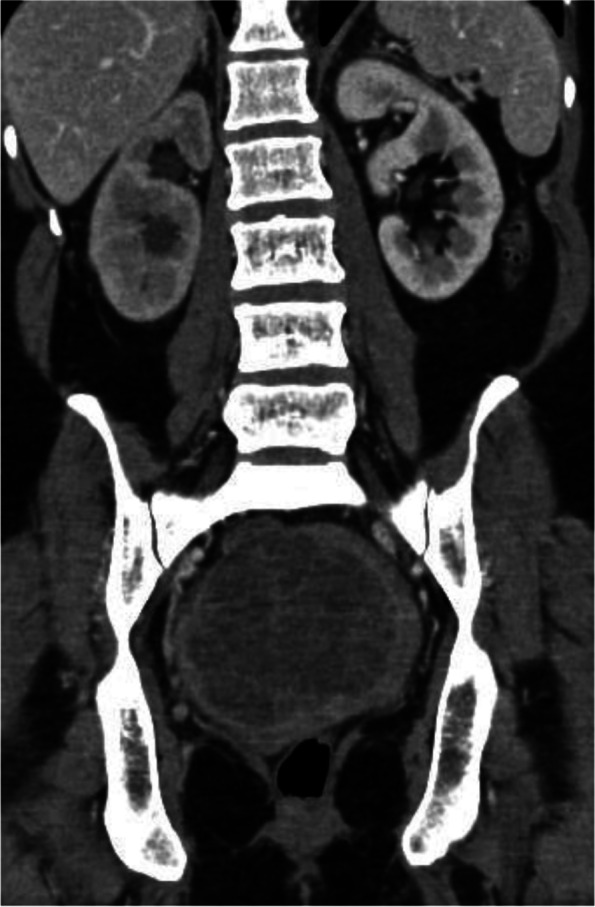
Coronal reformatted CT image with IV contrast in a portal phase. Large pelvic mass, well-defined, predominantly hypoattenuating, with many thin septa and some enhancing areas in the periphery and the centre of the lesion. The right kidney shows mild calyceal dilation and delayed and diminished cortical enhancement reflecting obstructive uropathy
